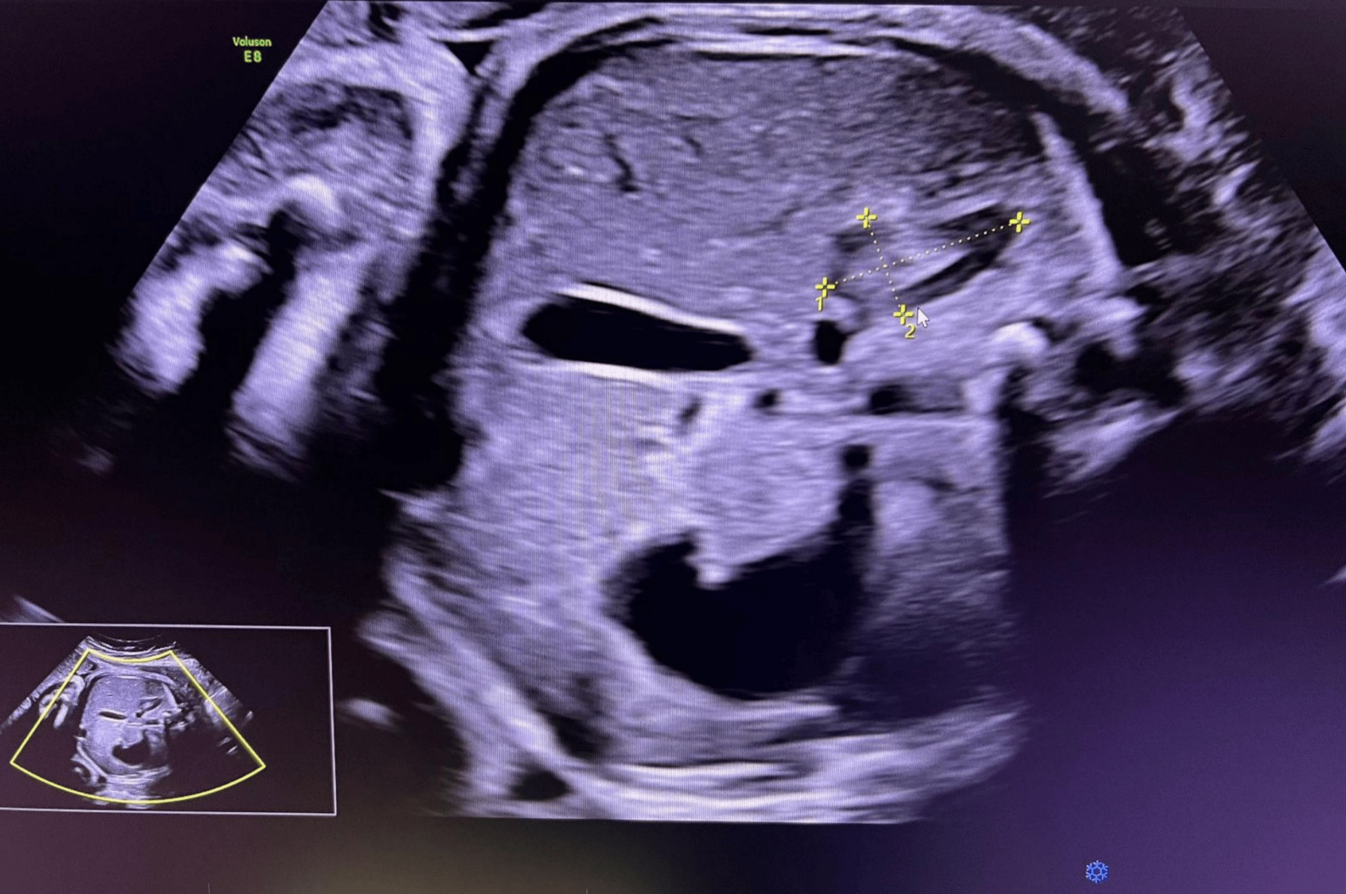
Axial ultrasound image of the fetal abdomen at 32 weeks’ gestation showing the right adrenal gland. The total gland length, width, and fetal zone dimensions are indicated
